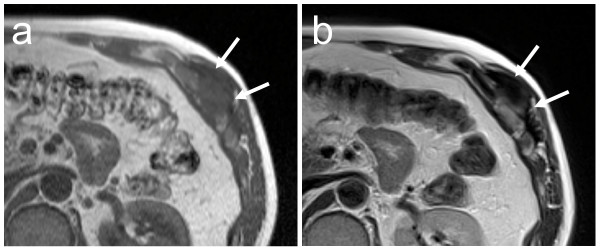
Axial MRI of the chest wall after treatment with tranilast. The tumor on T1-weighted image (a) is smaller than that seen on his first visit (shown in Figure 1(a)). The proportion of low-signal intensity area on T2-weighted image (b) is larger than that seen on his first visit (shown in Figure 1b). Arrows indicate the tumor.
